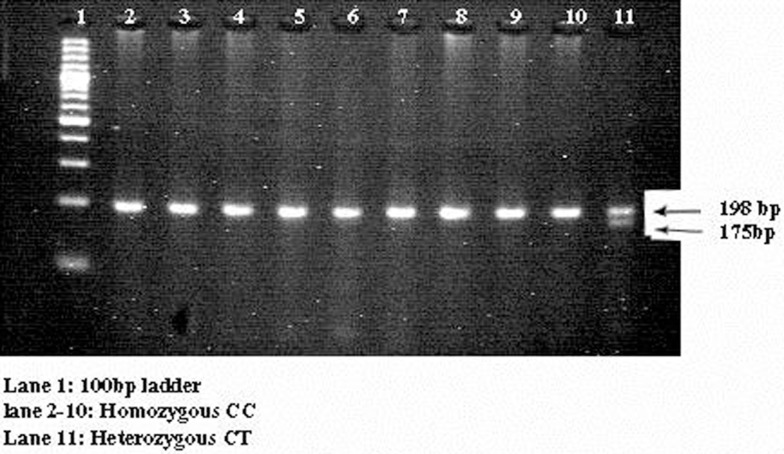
Three percent agarose gel consist of digested products of C677T mutation. It represents the 3% agarose gel picture. Lane 1 consists of 100 bp ladder. Lanes 2–10 consist of homozygous CC genotypes and lane 11 consists of heterozygous CT genotype.
